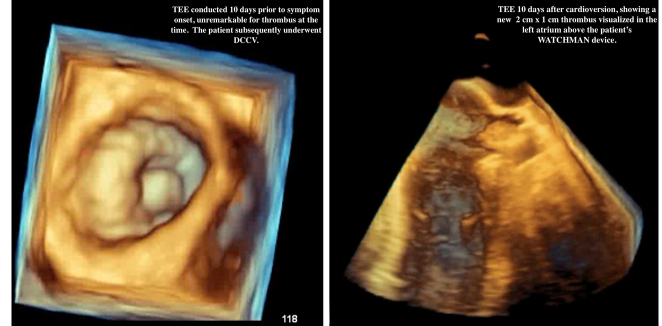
Transesophageal echocardiography images before cardioversion and after.
